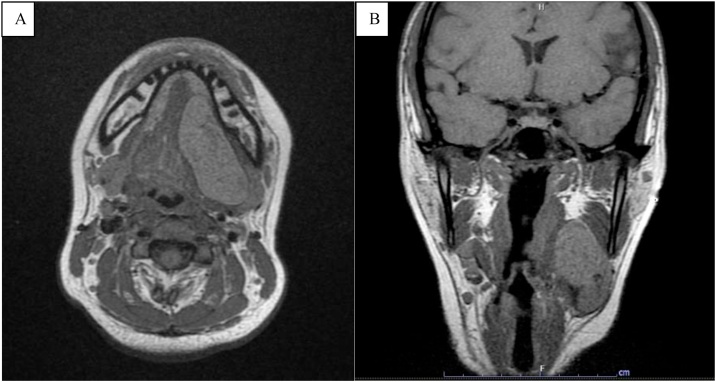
MRI of axial view (A) and coronal view (B) demonstrates the left sublingual epidemoid cyst extended posteriorly to the left parapharyngeal space.
